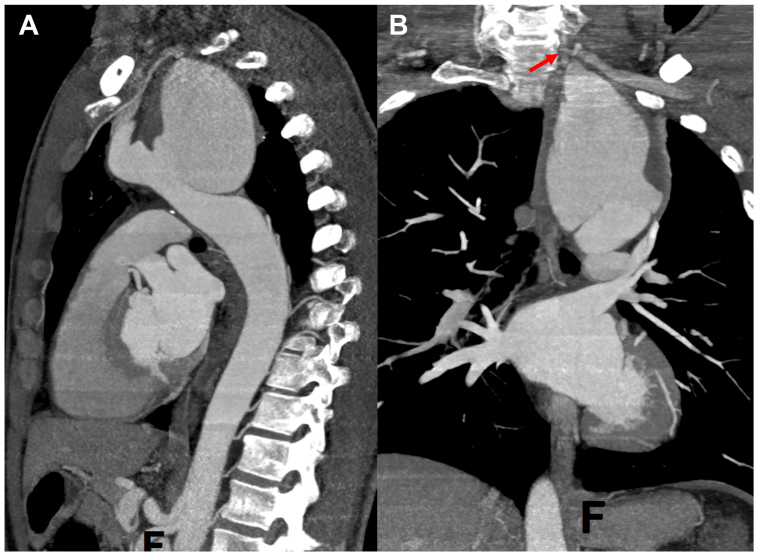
(A) Sagittal reconstruction of computed tomography angiography (CTA) demonstrating a giant intrathoracic pseudoaneurysm arising from the proximal left subclavian artery (LSA). (B) Coronal reconstruction showing the close proximity of the pseudoaneurysm to the origin of the left vertebral artery (*red arrow*).
